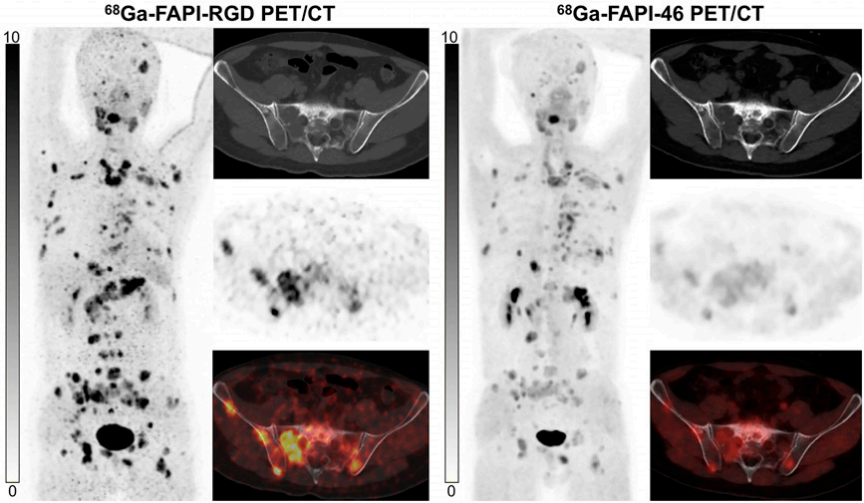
^68^Ga-FAPI-RGD PET/CT shows significantly higher radiotracer uptake than ^68^Ga-FAPI-46 in patient with metastatic thyroid cancer.
